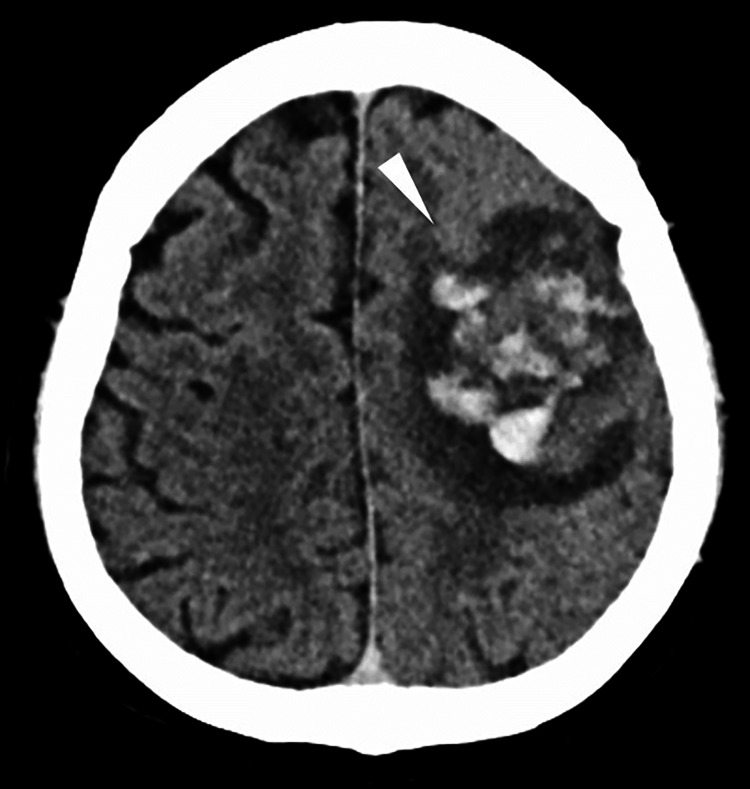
CT three days after admission Image showing intracerebral hemorrhage in the left frontal lobe (white arrowhead).
